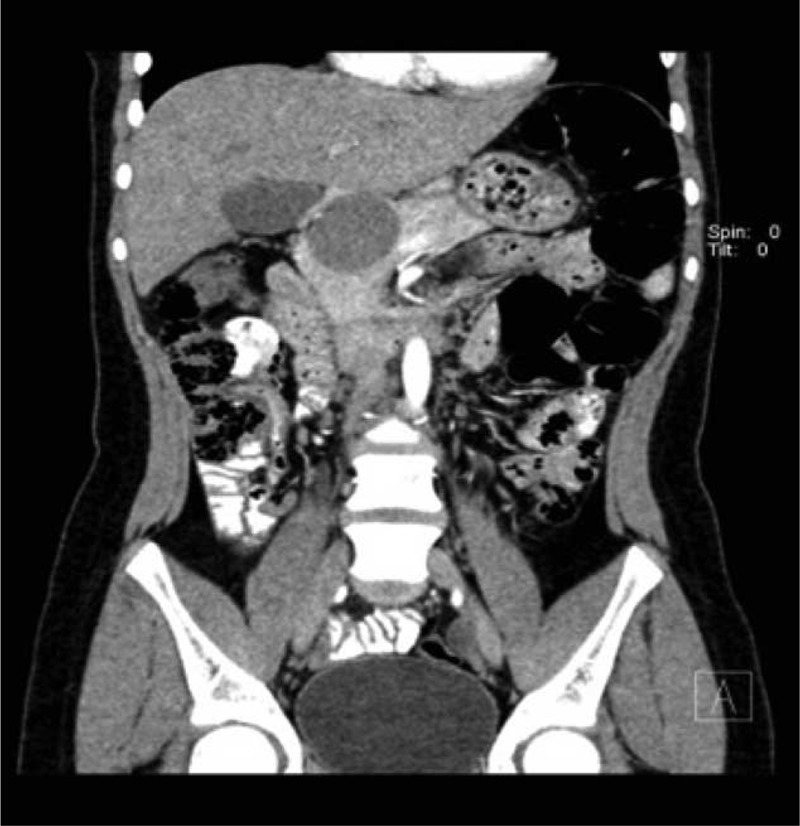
Computed tomography (CT) scan: Anterio-posterior AP reconstruction – tumor located in the head and body of the pancreas – visible margin of pancreatic head.
